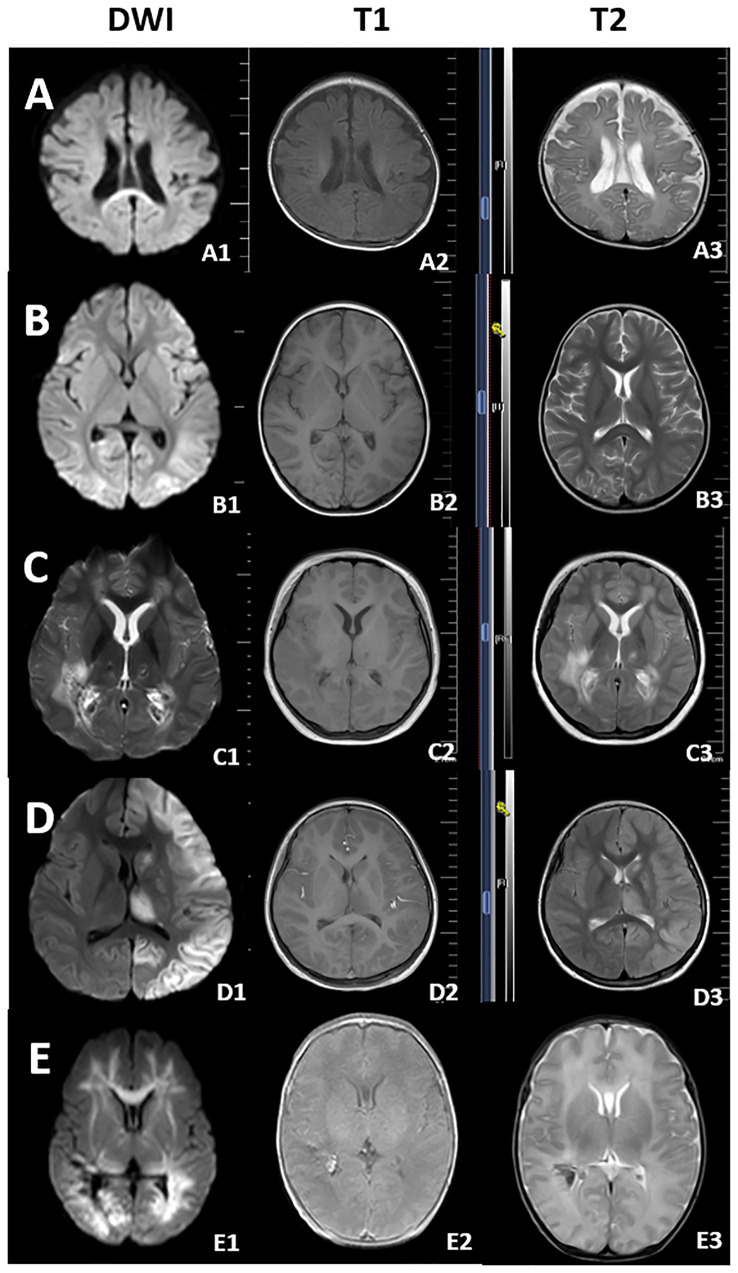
HEAD MRI of critically ill children infected with Omicron variant. (A) A 2-month-old boy with febrile seizures (For lung CT, see Supplementary Figures D1, D2). Cytotoxic edema was seen in the splenium of the corpus callosum. (B) A 28-month-old girl with febrile seizures. She had no obvious symptoms of respiratory system. Multiple cytotoxic edema lesions was seen in the brain. (C) A 11-year-old girl with febrile seizures. (D) A 9-year-old girl with status convulsion. She underwent shock and tracheal intubation and was discharged on the 8th day. (E) A 1-month-old boy, was admitted to the PICU at 39 weeks PMA. Head MRI shows extensive lesions in the brain.
